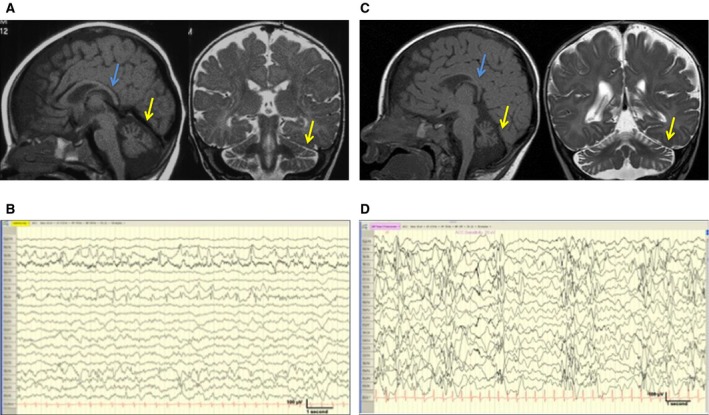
Radiologic and electrographic features. (A) MRI brain scan for patient 1, 7 months of age: Sagittal T_1_‐weighted and coronal T_2_‐weighted imaging showing generalized reduction in white matter bulk and marked cerebellar atrophy (yellow arrow) and corpus callosum thinning (blue arrow) with relative sparing of the pons. (B) Last EEG of patient 1, at 6 years of age, continued to show frequent epileptiform activity more prevalent over the posterior regions. (C) MRI brain scan of patient 2, age 6 months: Sagittal T_1_‐weighted and coronal T_2_‐weighted imaging shows marked cerebellar atrophy (yellow arrow), thinning of the corpus callosum (blue arrow), relative sparing of the pons, and reduction in white matter bulk. (D) EEG of patient 2, age 5 months: EEG showed frequent bursts of polymorphic high‐amplitude focal sharp delta/theta over both posterior temporal regions with a bias to the right.
